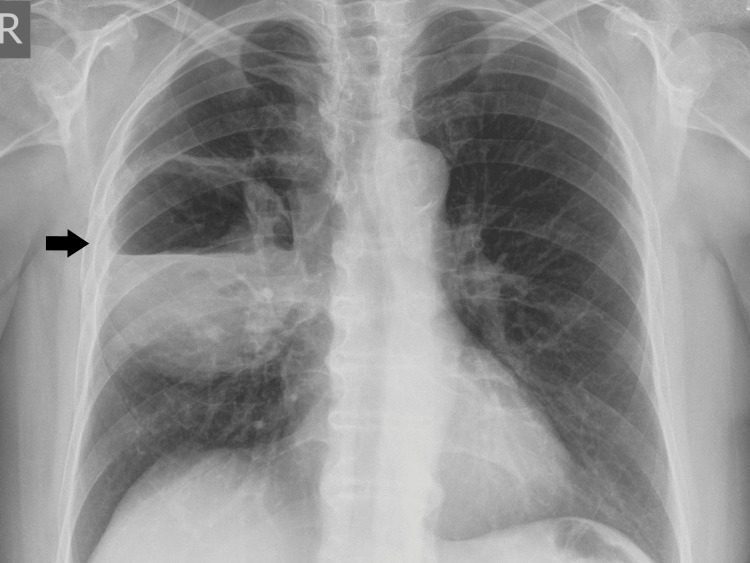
Chest x-ray, presenting an oval mass (arrow) in the right upper and middle lung lobes.
